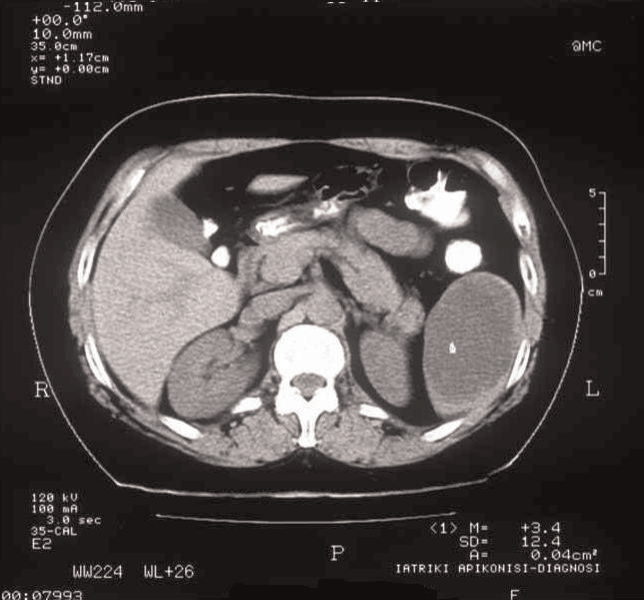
CT scan (2005): A 3 × 5 cm splenic cyst is seen located at the lower splenic pole.
